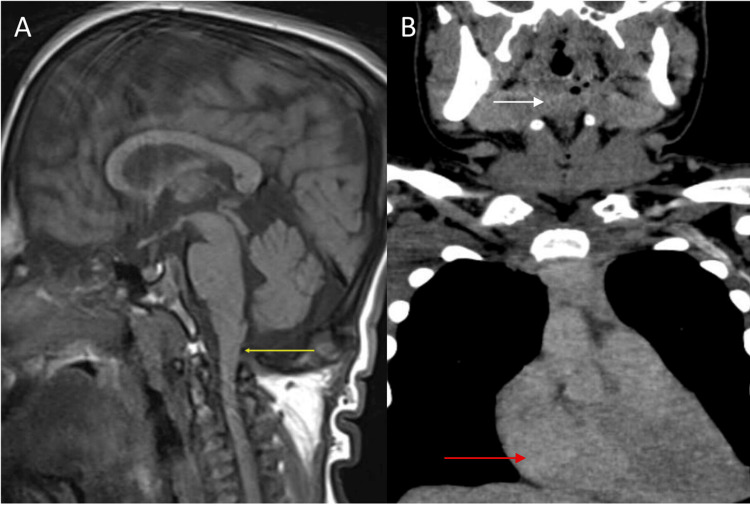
(A) Sagittal T1-weighted image (T1WI) shows crowding of the foramen magnum (yellow arrow) with the abnormal shape of cervical vertebrae, leading to spinal cord compression and compromise. (B) Coronal view shows soft tissue density (white arrow) causing oropharynx and hypopharynx narrowing. The red arrow indicates cardiomegaly.
